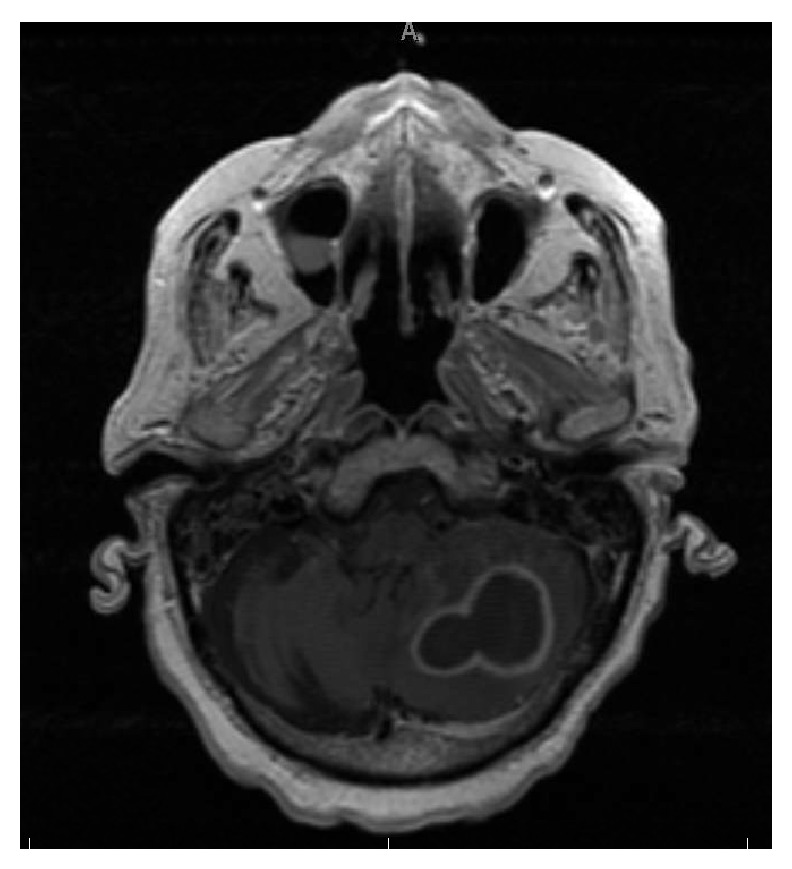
Magnetic resonance imaging of the brain showing ring enhancing lesion in the left cerebellum with mass effect on the fourth ventricle, one month after initial presentation.
